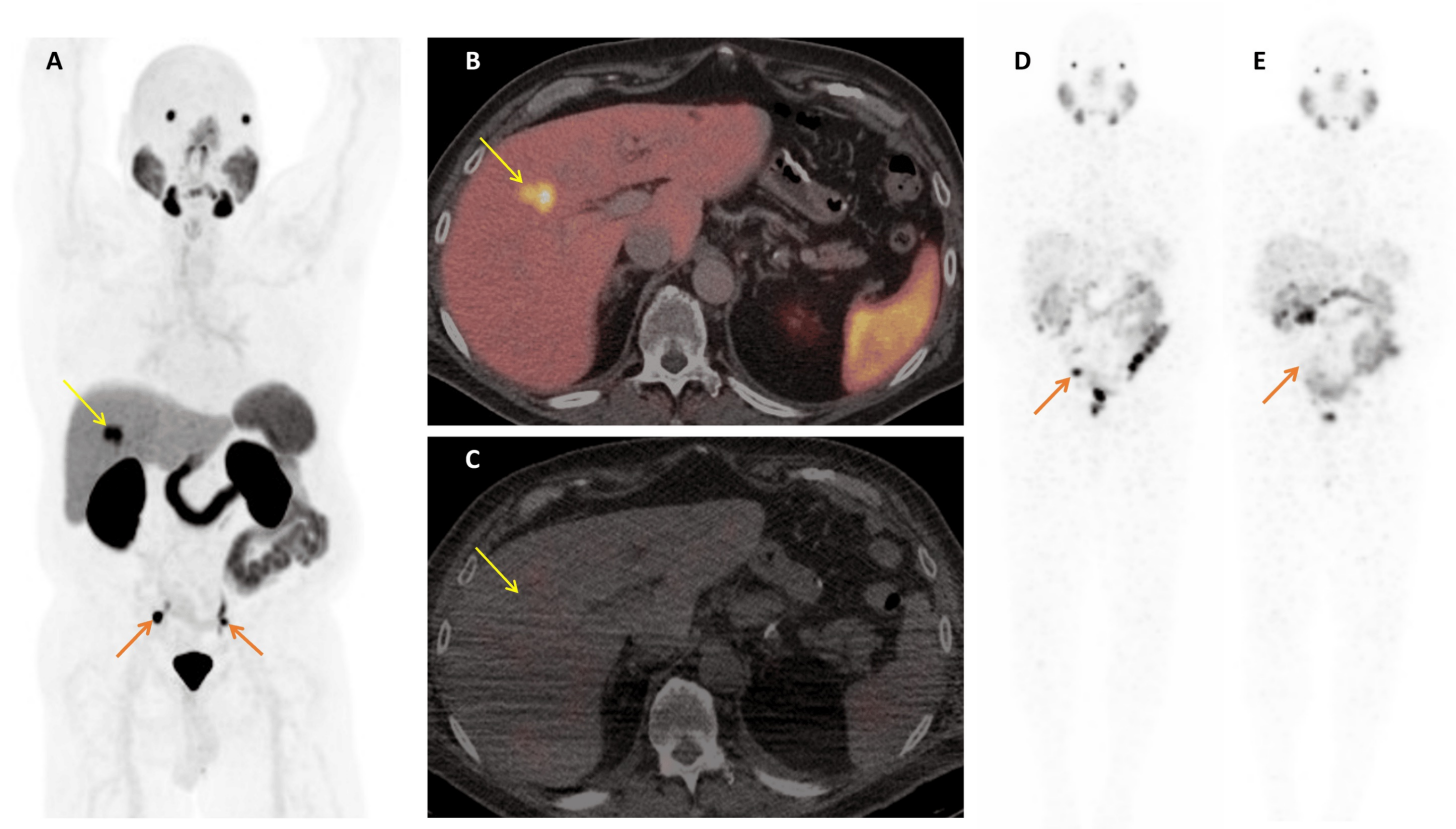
Initiation of prostate-specific membrane antigen (PSMA)-targeted radioligand therapy Pylarify (F-18 DCFPyL, a PSMA-targeted PET ligand) PET/CT showed a PSMA-positive liver lesion in the right hepatic lobe (SUVmax 14.6, yellow arrow) and PSMA-expressing right and left iliac lymph node metastases (orange arrows). Compared to prior imaging (not shown), the liver lesion had increased in size. After the first cycle of LuPSMA therapy, SPECT/CT demonstrated no significant long-term retention of LuPSMA in the liver lesion (yellow arrow), but persistent uptake was noted in the right iliac lymph node (orange arrow). Following the second therapy cycle, the right iliac node showed a marked decrease in PSMA expression and size on SPECT/CT (orange arrow).
